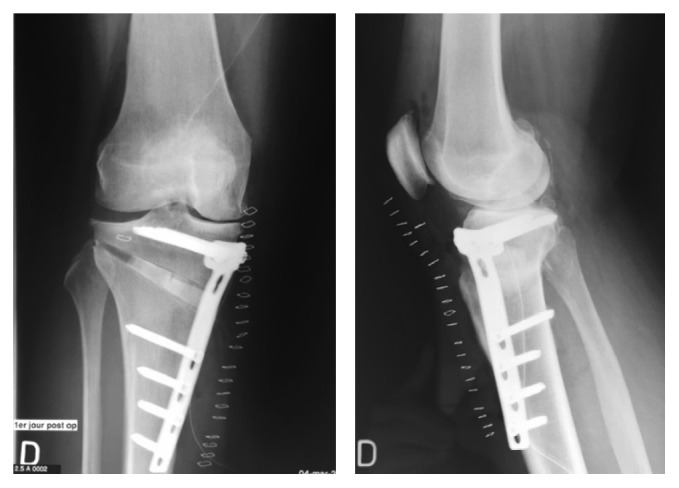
Postoperative X-rays showing fracture of the lateral cortex at the osteotomy site.
